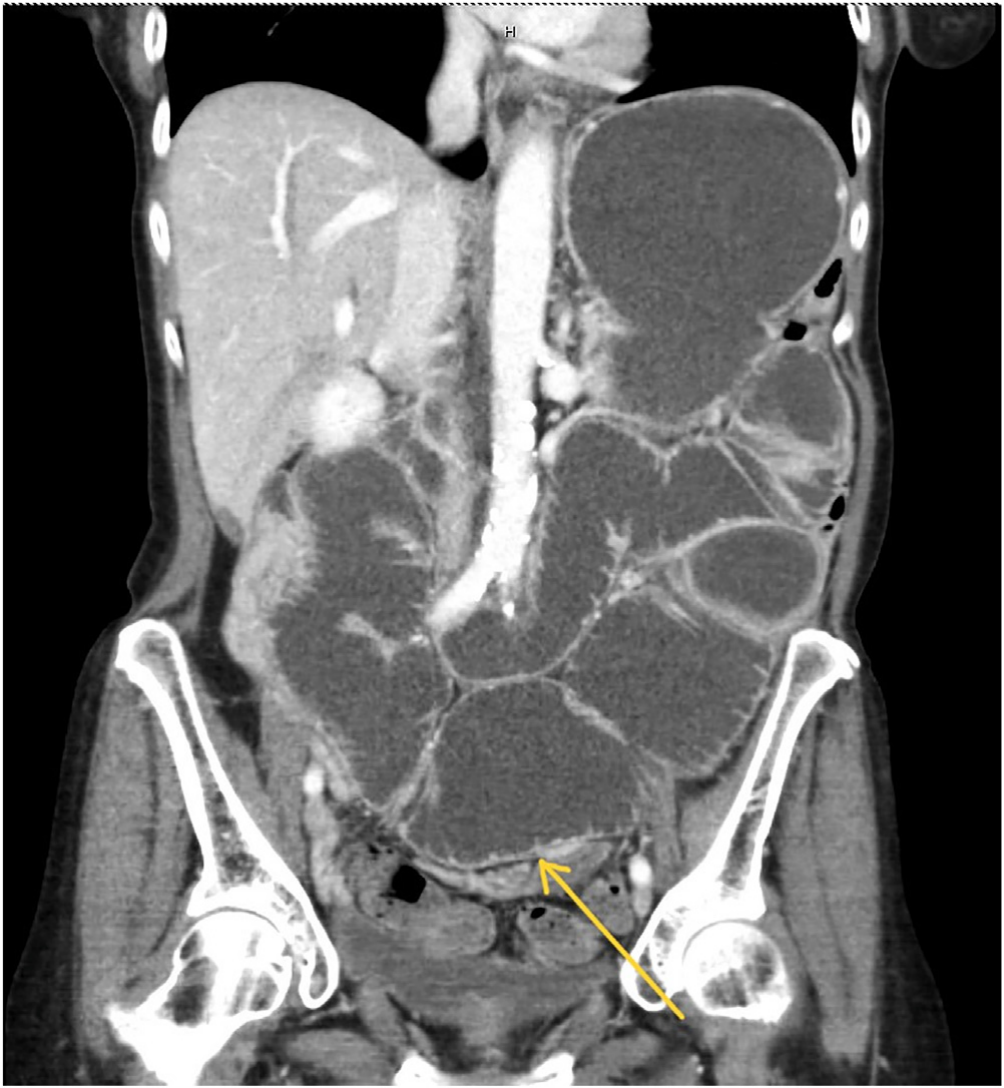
Abdominal/pelvic CT image demonstrating small-bowel obstruction. The *yellow arrow* points to the opposition between the ileum and the rectosigmoid colon.
